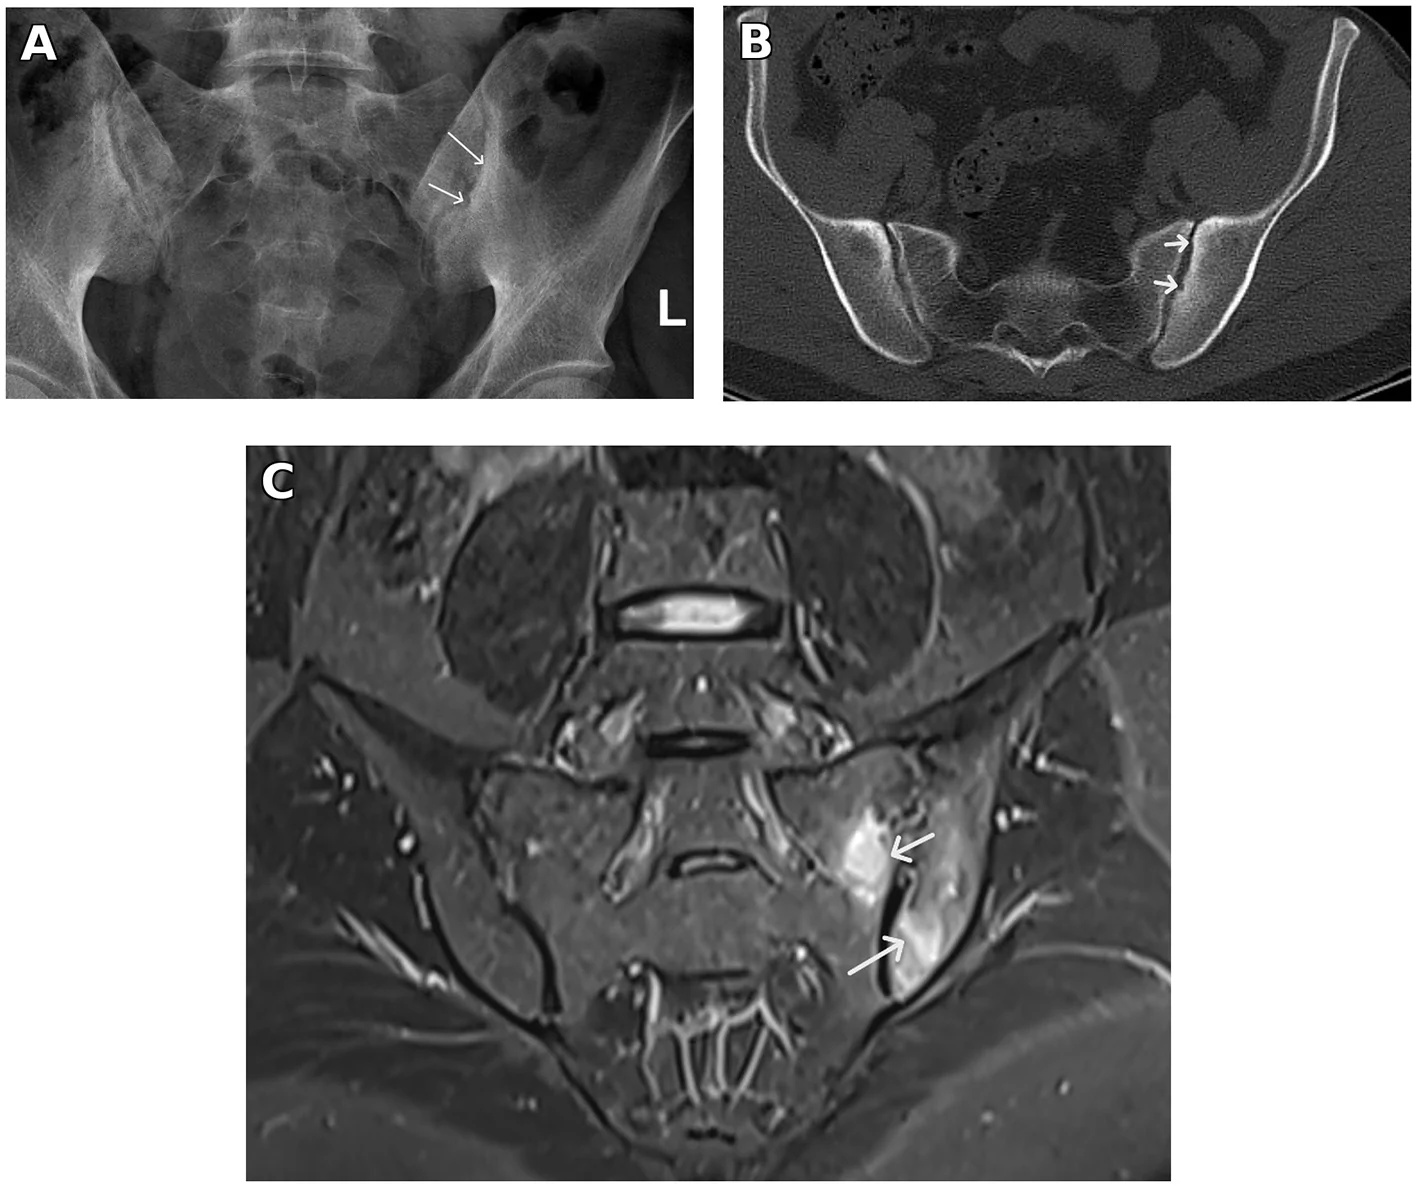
Baseline sacroiliac imaging. (A) Conventional sacroiliac-joint radiograph. White arrows indicate mild left-sided subchondral sclerosis and subtle articular-surface irregularity; the joint spaces remain preserved, without ankylosis, and definite radiographic sacroiliitis was not established. (B) Axial bone-window CT image. White arrows indicate subchondral sclerosis and focal articular-surface irregularity at the left sacroiliac joint, suggestive of possible early erosive change; the joint spaces remain preserved, without ankylosis. (C) Baseline semicoronal STIR MRI. White arrows indicate prominent subchondral bone marrow edema involving the iliac and sacral aspects of the left sacroiliac joint on the displayed slice. Additional inflammatory lesions were present bilaterally on adjacent slices, and the overall examination met the 2009 ASAS-OMERACT definition of active sacroiliitis on MRI.
